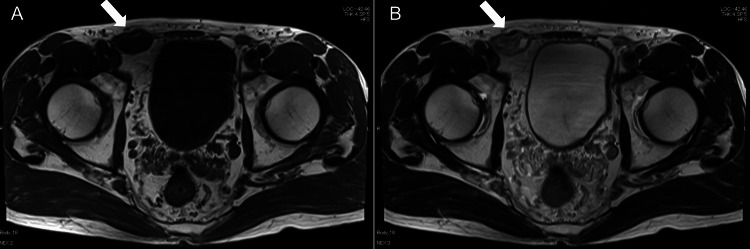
Magnetic resonance imaging of the groin The T1-weighted image (A) reveals a low-signal-intensity mass (white arrow) along the right spermatic cord, while the T2-weighted image (B) shows high signal intensity (white arrow). These signal characteristics suggest a cystic lesion.
